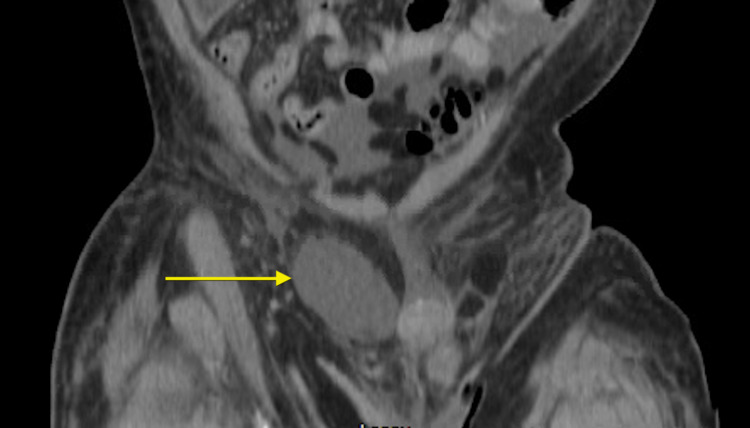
Coronal CT imaging obtained at the time of penile and scrotal metastatic presentation showing nodular mesenteric and peritoneal infiltration and thickening consistent with peritoneal carcinomatosis and a large mass present in the right hemiscrotum. CT: computed tomography
